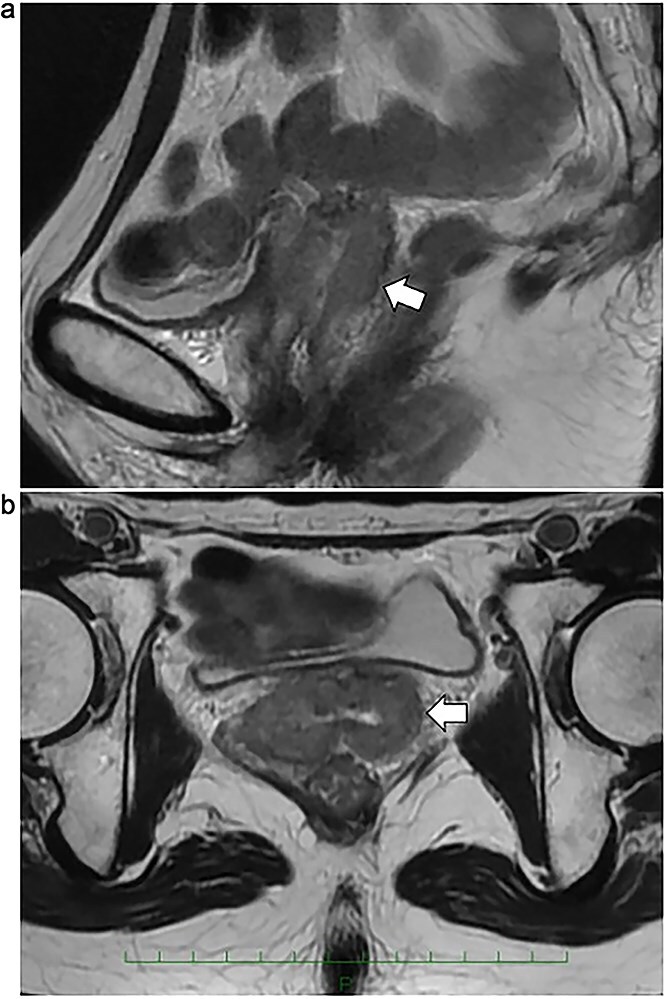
(a) Sagittal *T*_2_-weighted MRI prior to reirradiation. A recurrent tumor (arrow) is visualized along the vaginal cuff, exhibiting contact with the adjacent small bowel. Adhesion between the tumor and bowel was suspected based on imaging. (b) Axial *T*_2_-weighted MRI demonstrating circumferential thickening of the recurrent lesion (arrow) along the entire vaginal wall. No evidence of pelvic lymph node or distant metastasis was identified on MRI, CT, or PET-CT.
